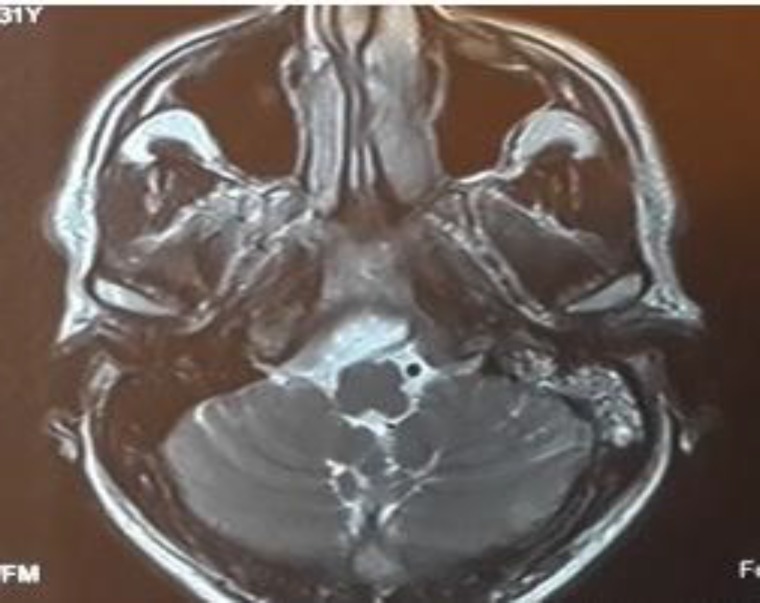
Brain MRI axial T2WI without Gad showing left mastoid air cell opacification and involvement of the left petrous apex
